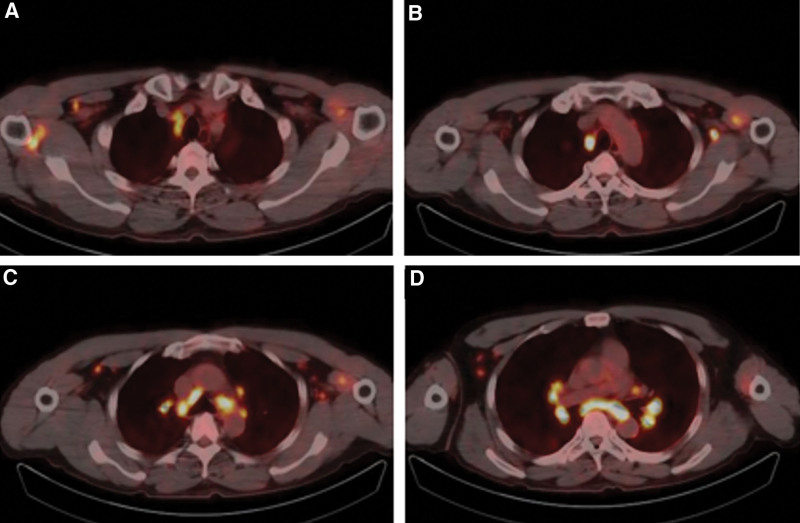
18F-FDG-PET imaging after five cycles demonstrating bilateral supraclavicular, mediastinal, bilateral hilar, bilateral axillary lymph nodes were enlarged with hypermetabolism, the right interlobar pleural nodules were slightly higher, the subpleural metabolism of both lungs was slightly higher. FDG = fluorodeoxyglucose, PET = positron emission tomography.
